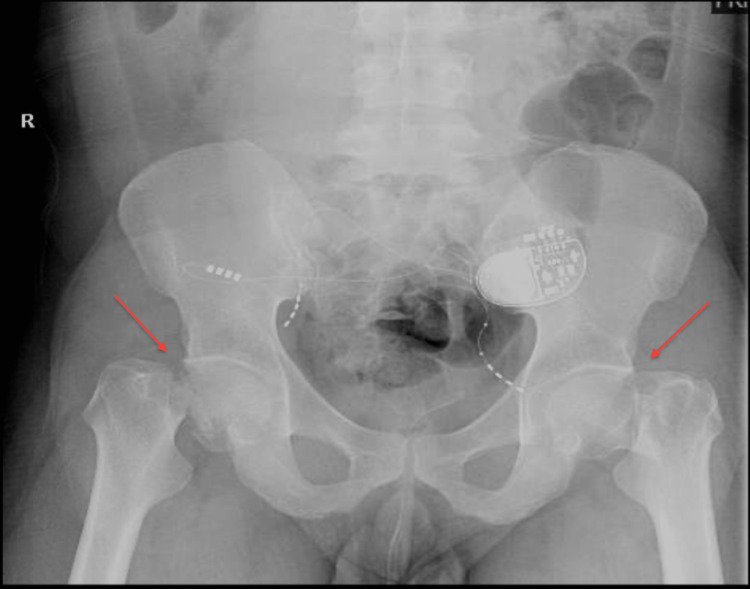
Bilateral neck of femur fracture x-ray
